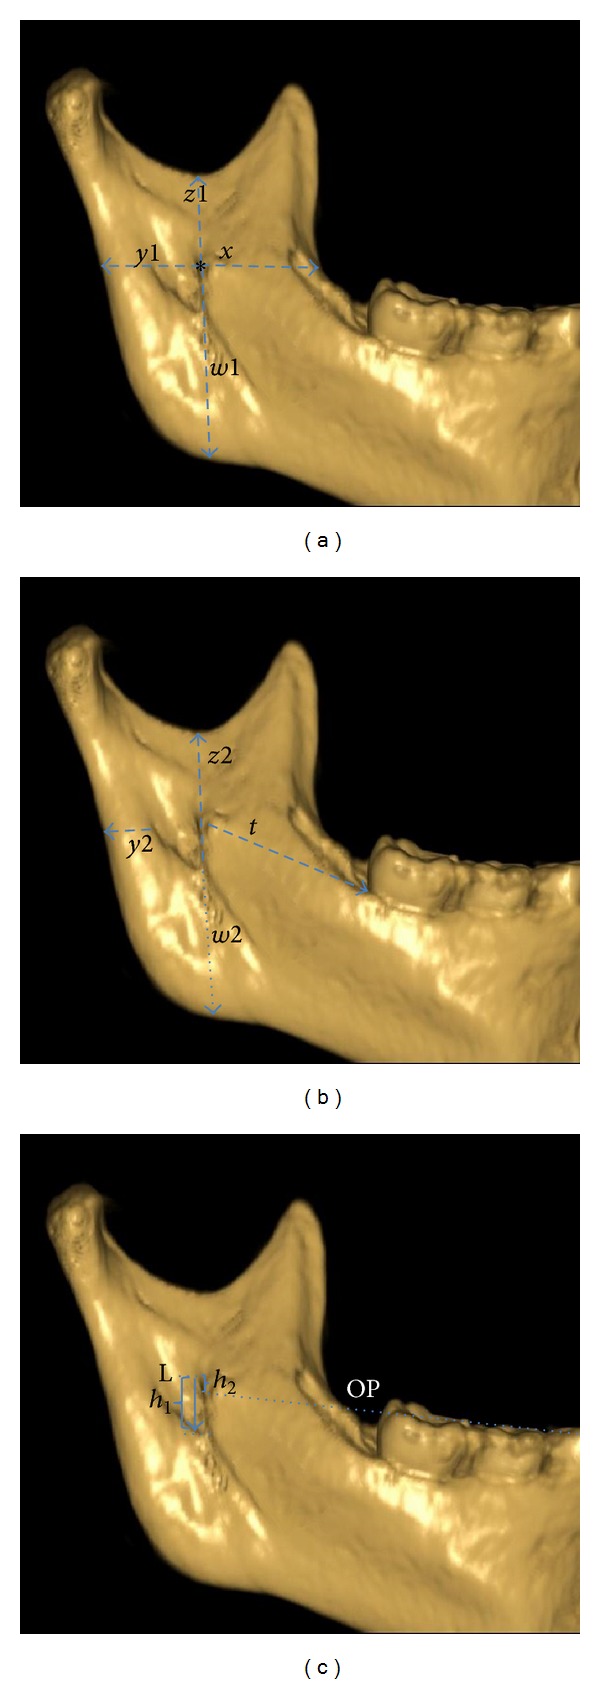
Three-dimensional CBCT image of the internal surface of the mandible showing the measurement for localizing the position of the lingula, entrance of mandibular foramen from the various landmarks. The “*x*” measurement indicated the distance in millimetres found between the most anterior part of the mandibular lingula points to the anterior border of the mandibular ramus in a straight horizontal line; the “*y*” measurement represented the distance between the most posterior part of the mandibular lingula and foramen points to the posterior border of the mandibular ramus in the same horizontal orientation; the “*w*” measurement determined the distance between the lower point of the mandibular foramen and lingula points to the mandibular base in a vertical straight line; the “*t*” measurement determined the distance most anterior part of the mandibular lingula points to the alveolar socket of first mandibular molar tooth; the “*z*” measurement determined the distance between the same initial points as for the *w* distance to the lower point of the sigmoid notch. The horizontal distances were measured parallel to the occlusal plane (OP) of the molars, whereas the vertical distances were measured perpendicular to the occlusal plane of the molars. The ratio of (*x*) to (*x* + *y*1) was also calculated and used as an additional guide to localize the lingula [21]. Vertical distance from the tip of the lingula to the lower border of the mandibular foramen was measured as height of the lingula (*h*
